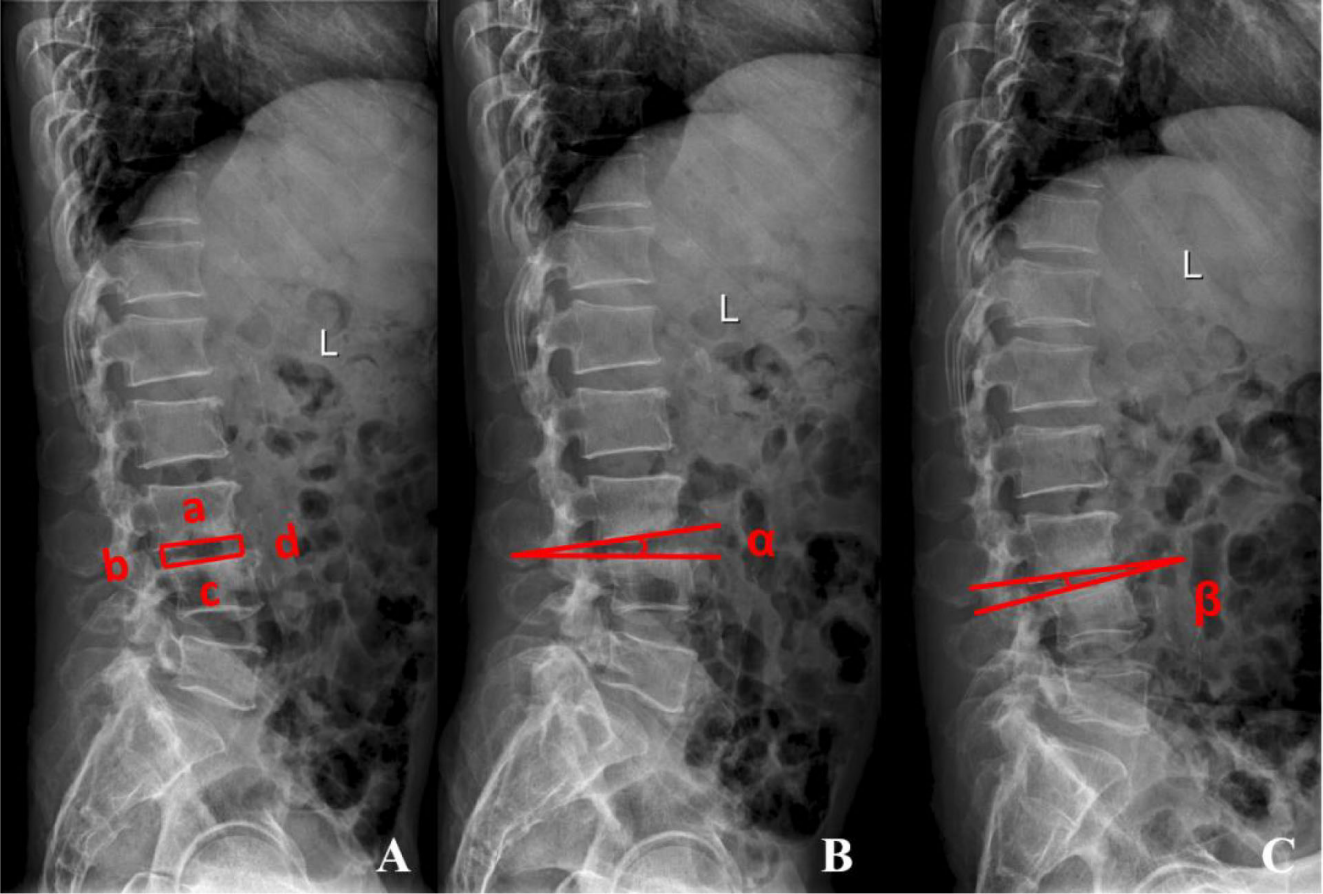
Radiographic index measurement methods: (A) Intervertebral Height Index (IHI)= (b + d)/(a + c)*100%. (B, C) Segmental Range of Motion(sROM)= |α - β| if angles in same direction; α + β if angles in opposite directions. ①Intervertebral Height Index: Measurement of affected intervertebral space height on preoperative and postoperative lateral radiographs. (a. Width of the inferior endplate of the upper vertebra; b. Height between posterior vertebral body lines; c. Width of the superior endplate of the lower vertebra; d. Height between anterior vertebral body lines.) ②Segmental Range of Motion: The intervertebral angle at the affected level is measured on preoperative and postoperative flexion and extension radiographs.
